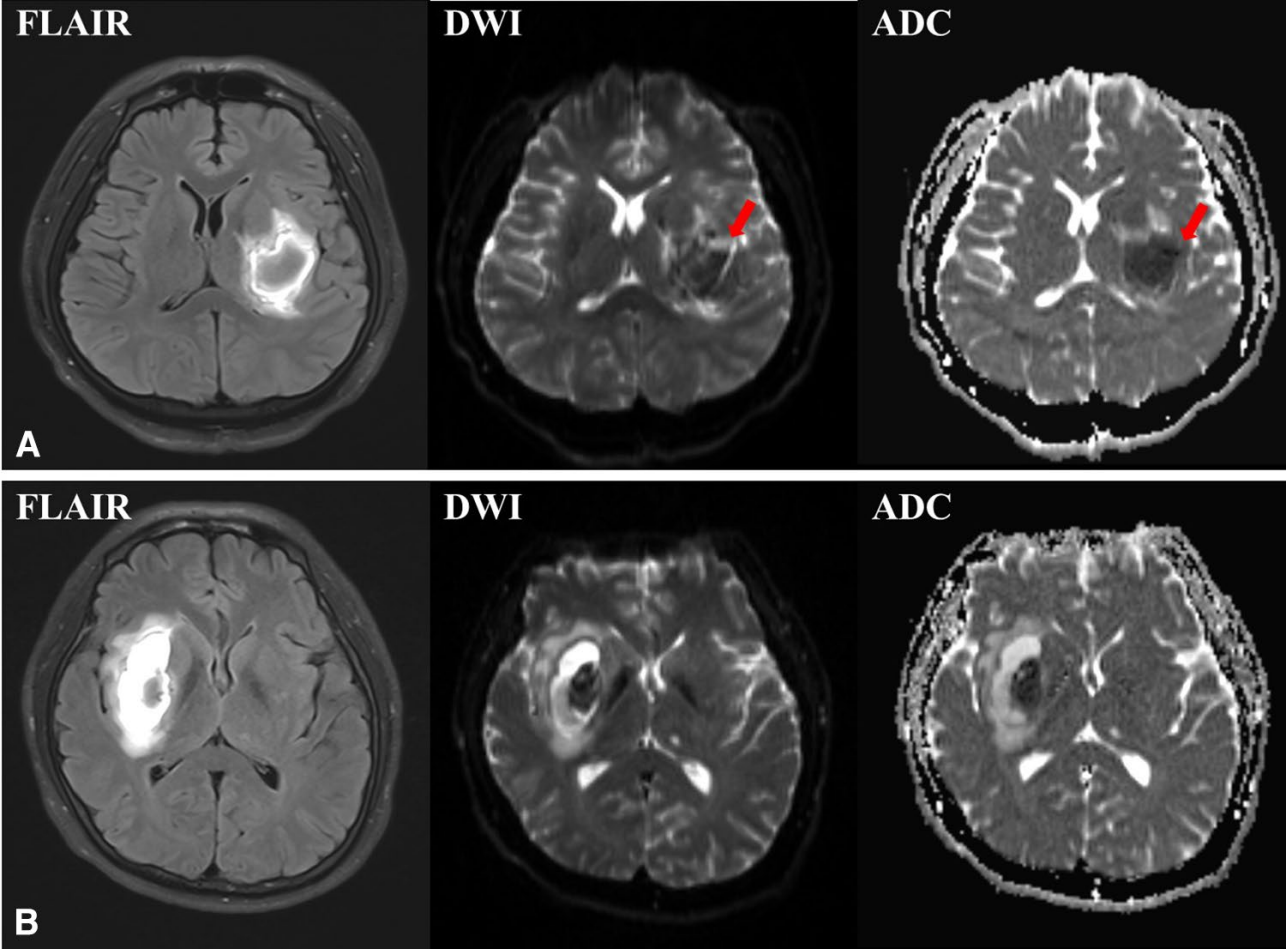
Example of cytotoxic edema (CE) on magnetic resonance imaging. a A patient with CE who developed a poor functional outcome. b A patient without CE who had a favorable outcome. ADC apparent diffusion coefficient, DWI diffusion-weighted imaging, FLAIR fluid-attenuated inversion recovery
